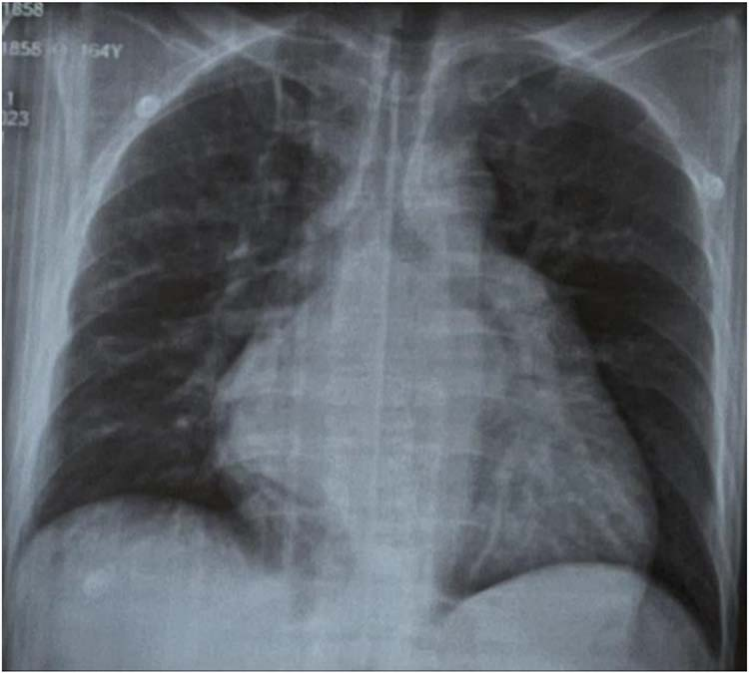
Chest radiography revealed cardiomegaly with radiological signs of pulmonary arterial and venous hypertension and biatrial enlargement.
